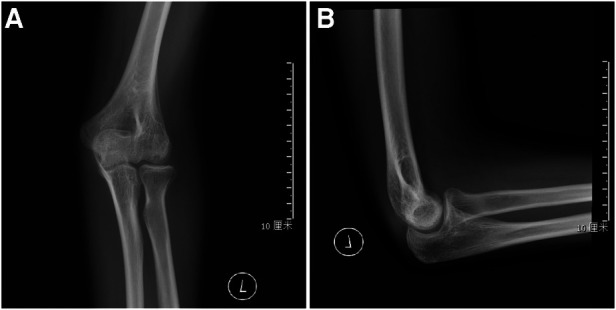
Follow-up: lateral (A) and AP radiographs (B) after surgery at the final follow-up.
